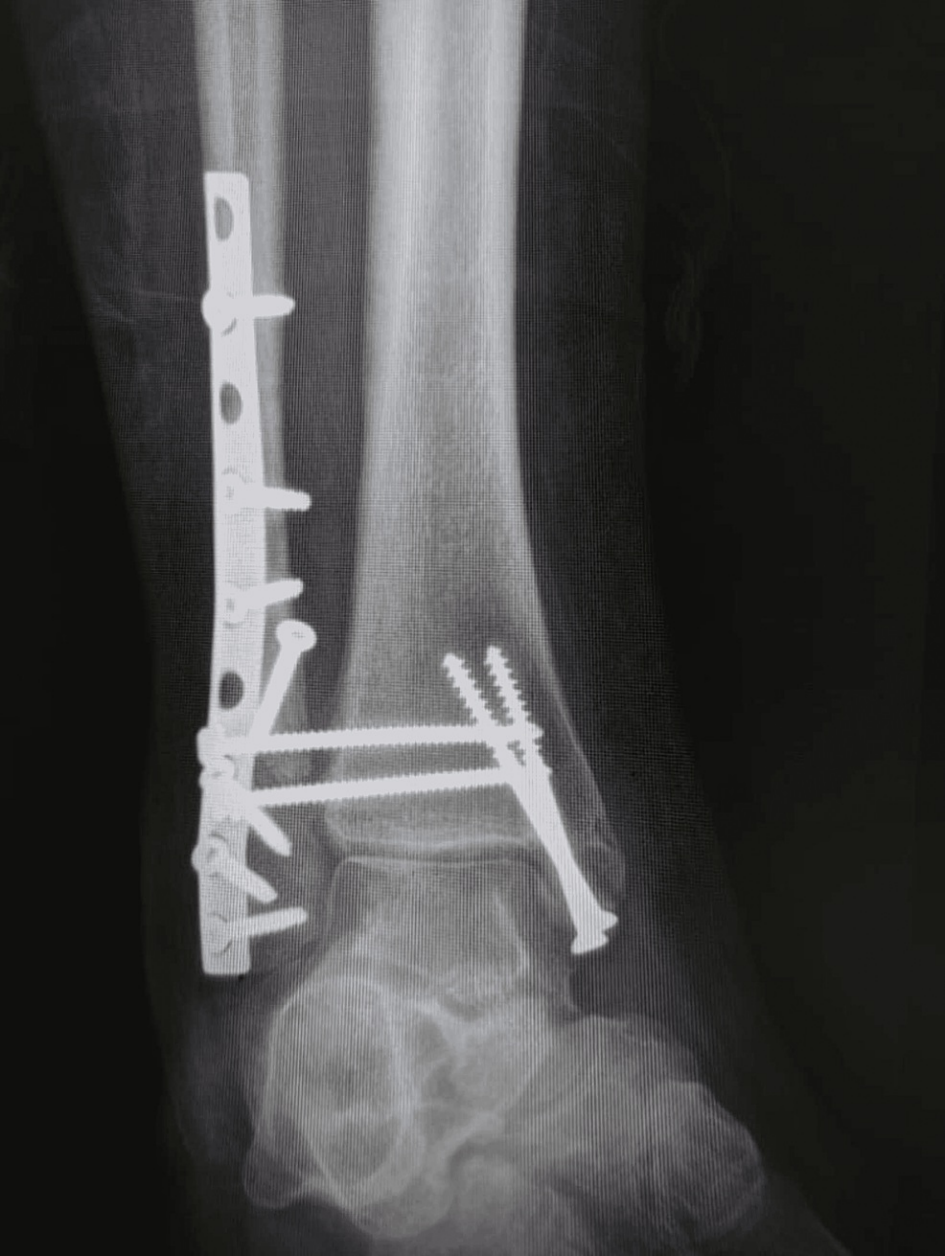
Follow-up radiograph anterior-posterior (AP) view
